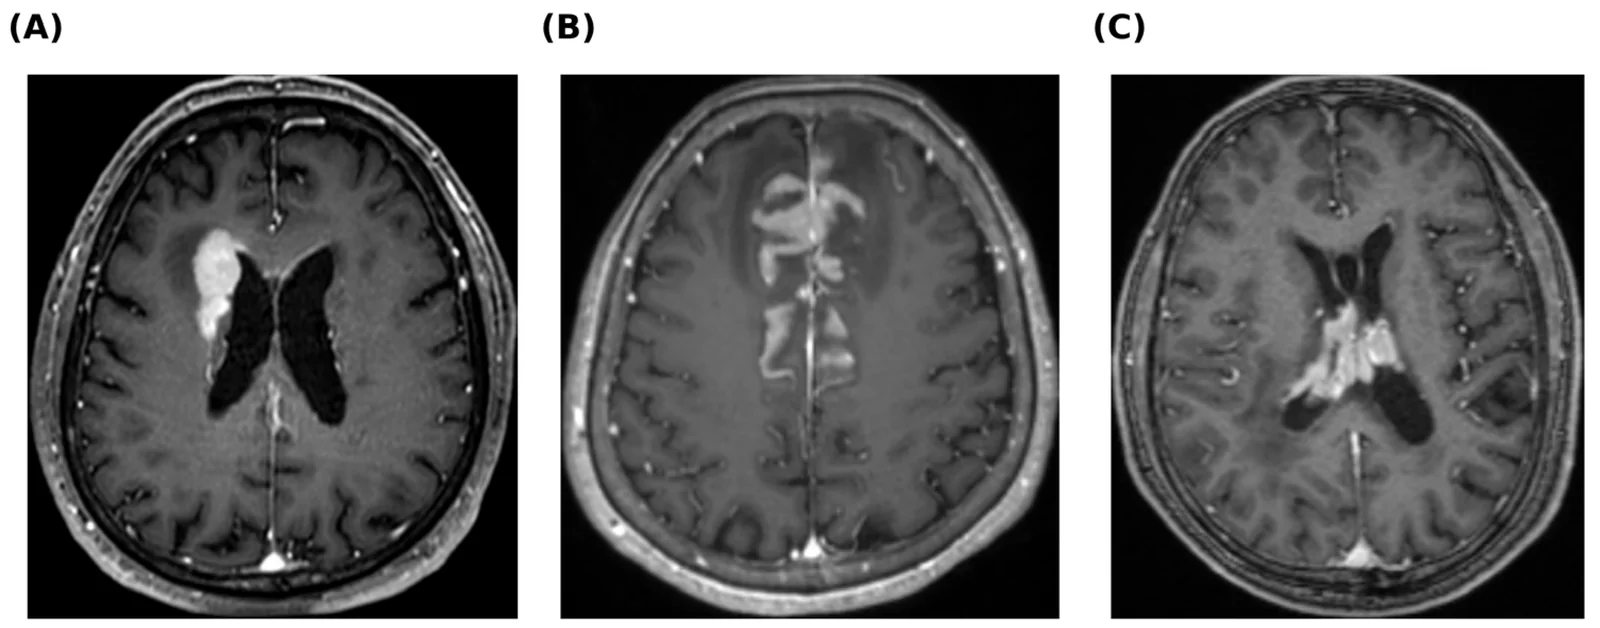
Representative magnetic resonance imaging (MRI) findings in primary central nervous system lymphoma. Axial contrast-enhanced T1-weighted MR images. (A) Curvilinear contrast enhancement along the right lateral ventricular wall, consistent with subependymal enhancement. (B) Linear enhancement along the leptomeningeal surfaces of the bilateral frontal lobes, consistent with leptomeningeal spread. (C) Bilateral enhancing masses within the lateral ventricular cavities, consistent with ventricular involvement.
